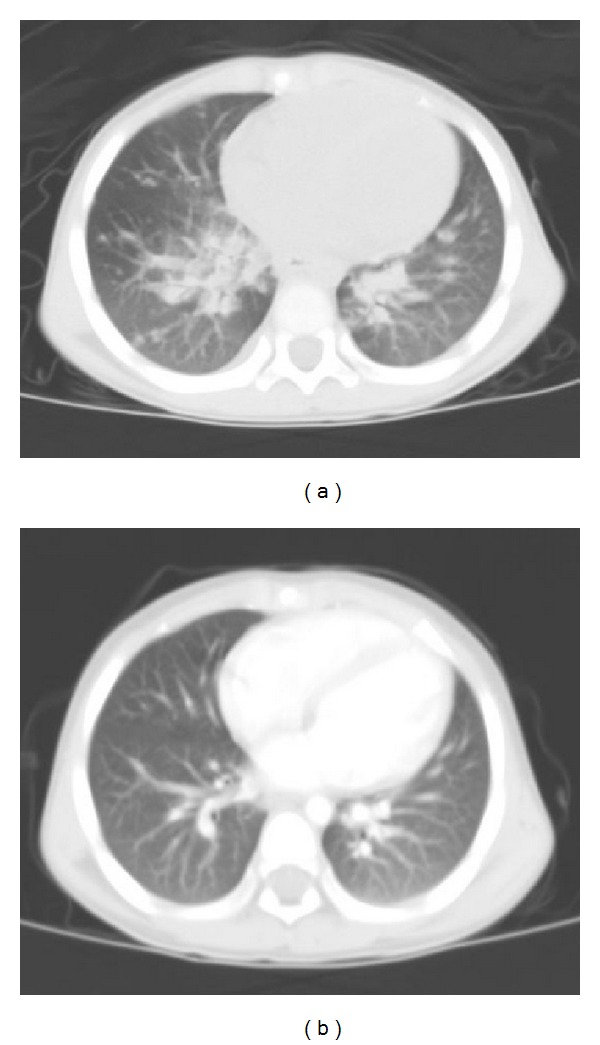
Thoracic CT reveals reticulonodular shadows in the lung window (a); after five weeks of treatment, thoracic CT showed that the lesions of the lung were reduced (b).
